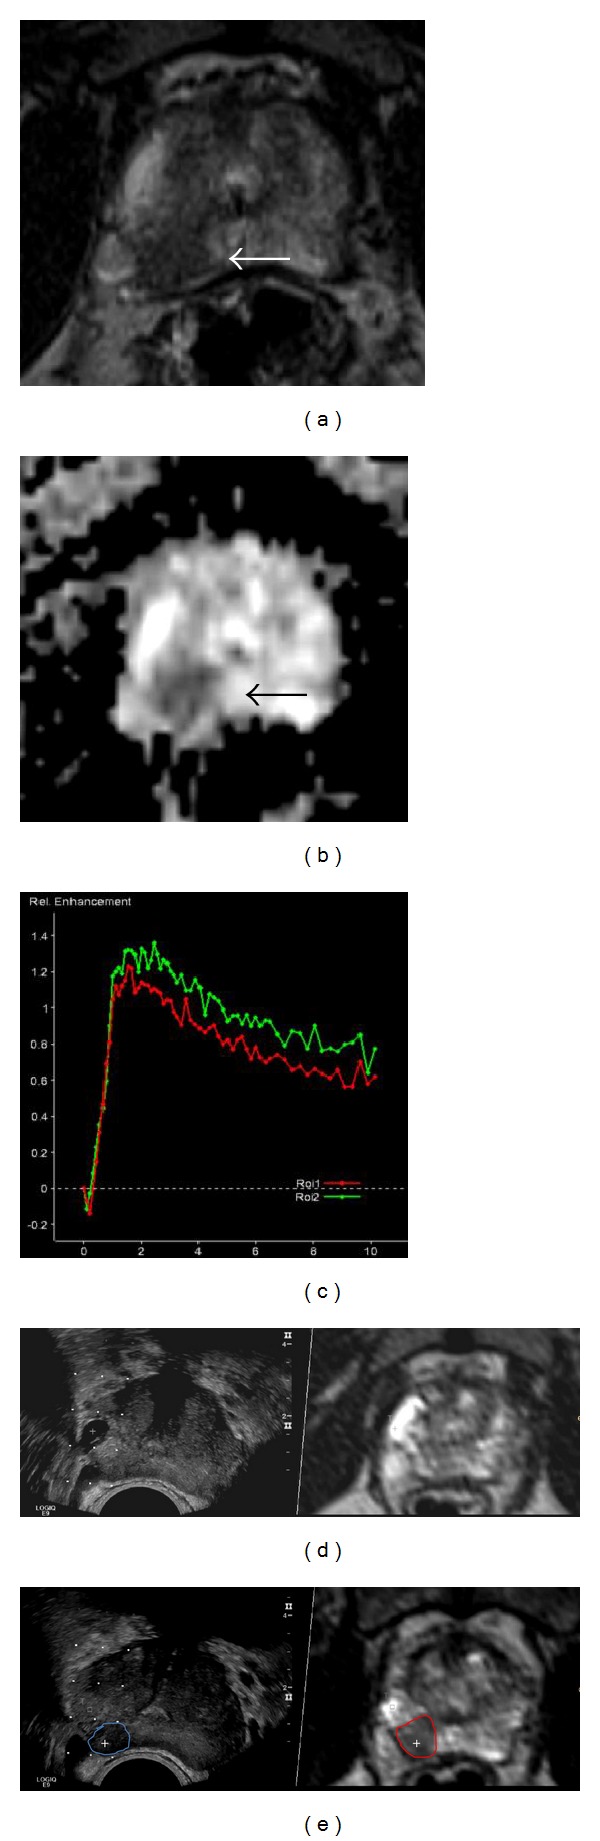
mpMRI-ultrasound image fusion: suspicious lesion (arrows) on T2W (a), on DWI with low ADC (b), and washout curve on DCE (c). Correlation of an anatomical landmark (cyst) for registration of *b*-mode ultrasound and SPACE 3D T2W-MRI (d). Target point (+) in the center of the suspicious lesion on *b*-mode ultrasound and SPACE 3D T2W-MRI (e). Note the slight deformation of the lesion (circle) on the ultrasound due to compression by the endorectal probe.
